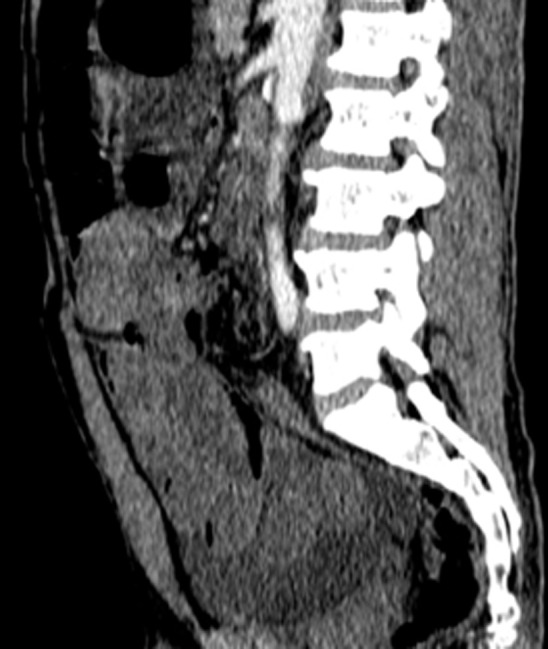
computed tomography in sagittal reconstructions, with injection of contrast product showing the distention of a sigmoid loop with a whirl sign
